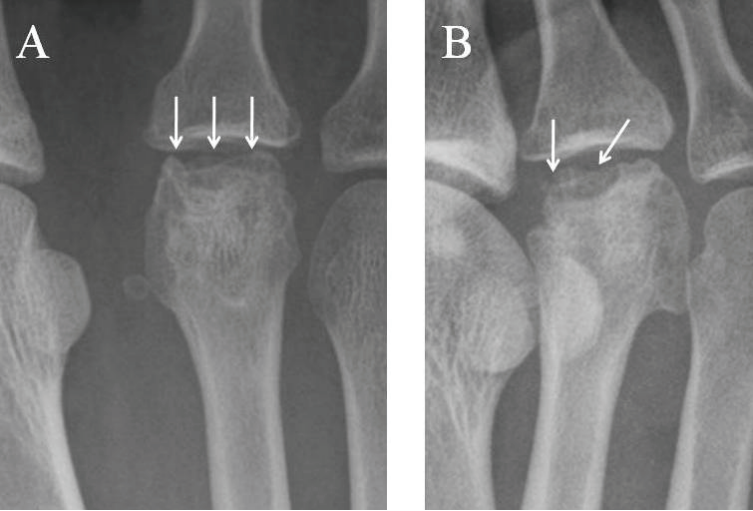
Radiograph of the patient's metatarsophalangeal (MTP) joint of the right second toe. (A) Anteroposterior and (B) oblique views of the MTP joint show that the second metatarsal head was flattened and sclerotic and also depict the irregularity of the joint surface of the head.
